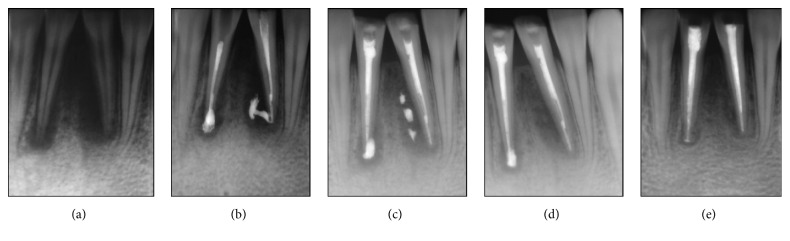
Bone defect and inflammatory resorption resulting from traumatic injury can be treated with CHISP. Inflammatory resorption at the root secondary to granulomas and infection in the pulpal space after avulsion were successfully treated using CHISP. (a) Preoperative radiograph of a clinical case of traumatic dental injury. (b) Intraoperative radiograph showing the injected paste through the root canal. (c) 10 days posttreatment exhibits fast material degradation. (d) Significant reduction of gap size and clear formation of bony tissue around the root of injured teeth. (e) Healing improvement and paste degradation after 60 days.
